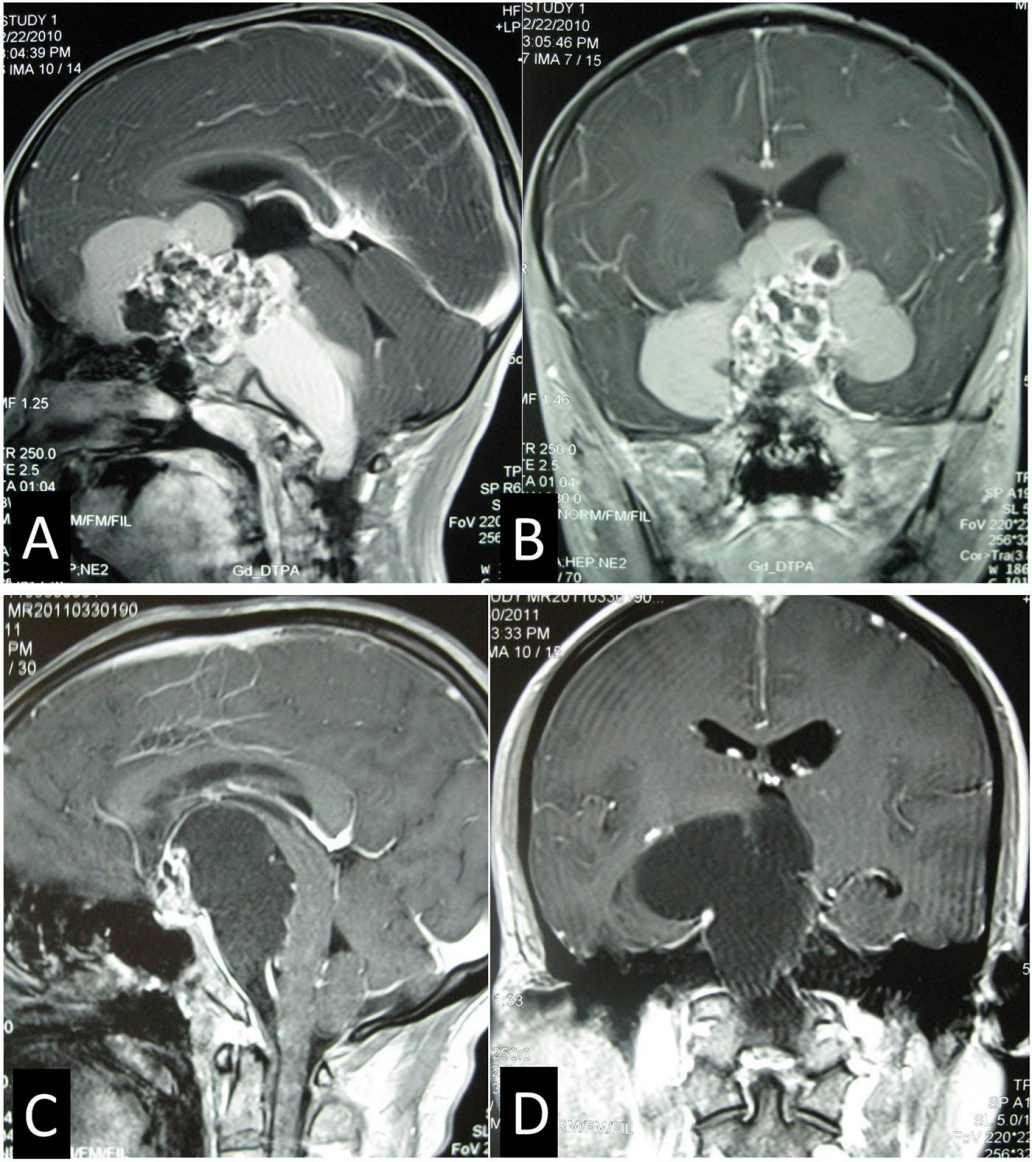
Examples of giant Suprasellar stalk CPs grow in roughly horizontal direction. (A and B) Sagittal (A) and Coronal (B) postcontrast T1-weighted MR images showing a giant Suprasellar stalk CP extends to the anterior fossa, sylvian fissure, the prepontine and posterior fossa. (C and D) Sagittal (C) and Coronal (D) postcontrast T1-weighted MR images showing a giant Suprasellar stalk CP extends to the prepontine and posterior fossa.
